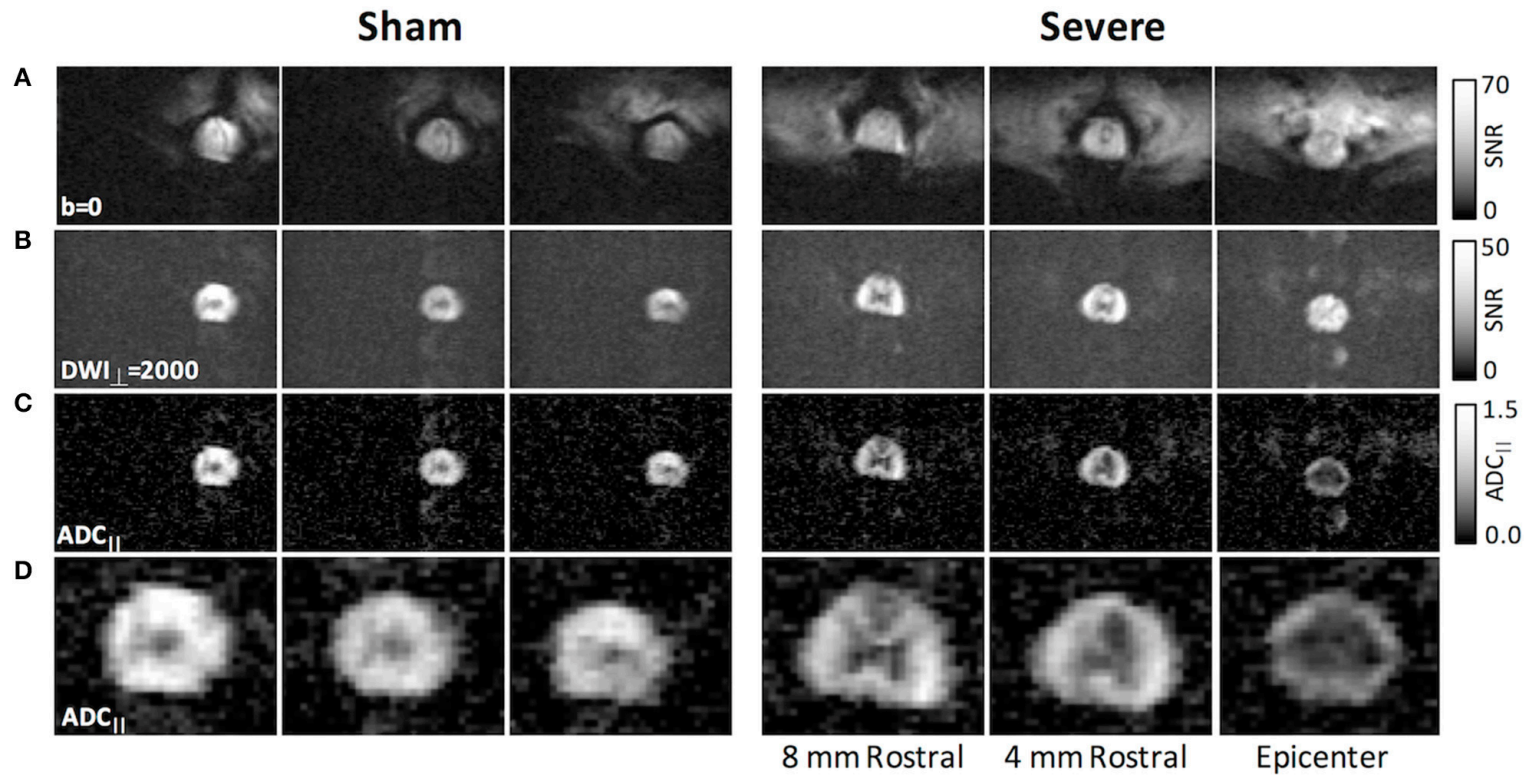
rFOV DWI in SCI with filter-probe SDE. Compared to the non-diffusion weighted images (A), the perpendicular diffusion-weighted images (B) were free from extraneous tissue signals, although some slight EPI ghosting was evident. The filtered ADC_||_ maps (C) reflected primarily the intra-axonal diffusivity. In an acute severe SCI (right), the ADC_||_ maps clearly demonstrated a reduction in the central region at the epicenter and in the dorsal columns rostral to the lesion. Magnification of filtered ADC_||_ maps are shown in (D).
